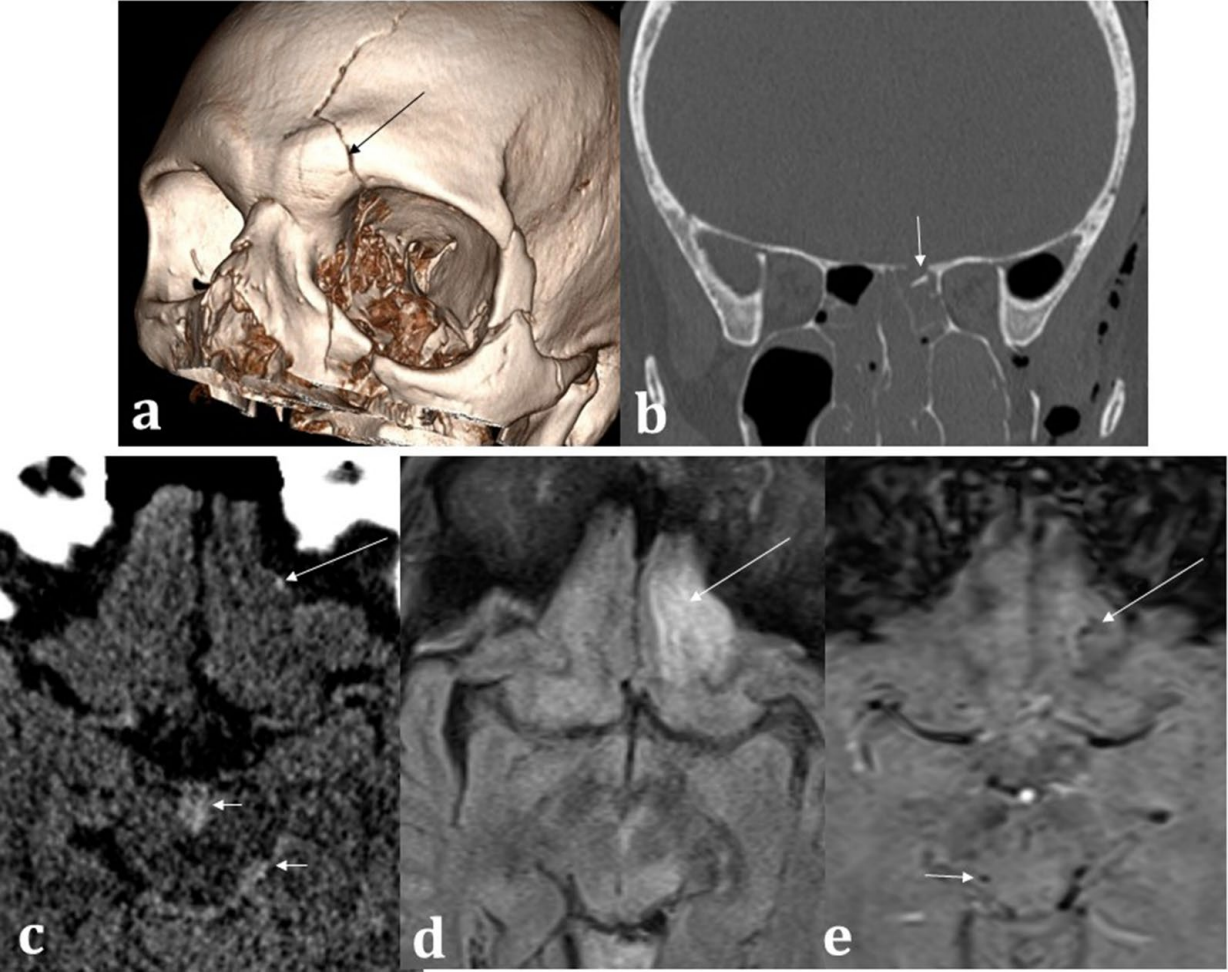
Trauma. 67-year-old male hit by a train. 3D CT reconstruction (a) demonstrates left-sided Le Fort III fracture complex and a calvarial fracture involving the frontal bone (arrowed). Coronal bone window CT (b) demonstrates an anterior cranial fossa fracture (arrowed) placing the patient at risk of inferior frontal lobe injury. Axial soft tissue window CT (c) confirms subtle punctate haemorrhage at the inferior frontal lobe (long arrow) as well as interpeduncular and ambient cistern subarachnoid haemorrhage (short arrows). The MRI axial FLAIR sequence (d) demonstrates a corresponding area of abnormal left inferior frontal lobe signal involving the olfactory sulcus (arrowed) with microhaemorrhages on the SWI (e) sequence (long arrow). Additional microhaemorrhages within the midbrain are consistent with grade III diffuse axonal injury (short arrows), and the aforementioned cisternal subarachnoid blood is also seen. The patient unfortunately passed away from related traumatic injuries
